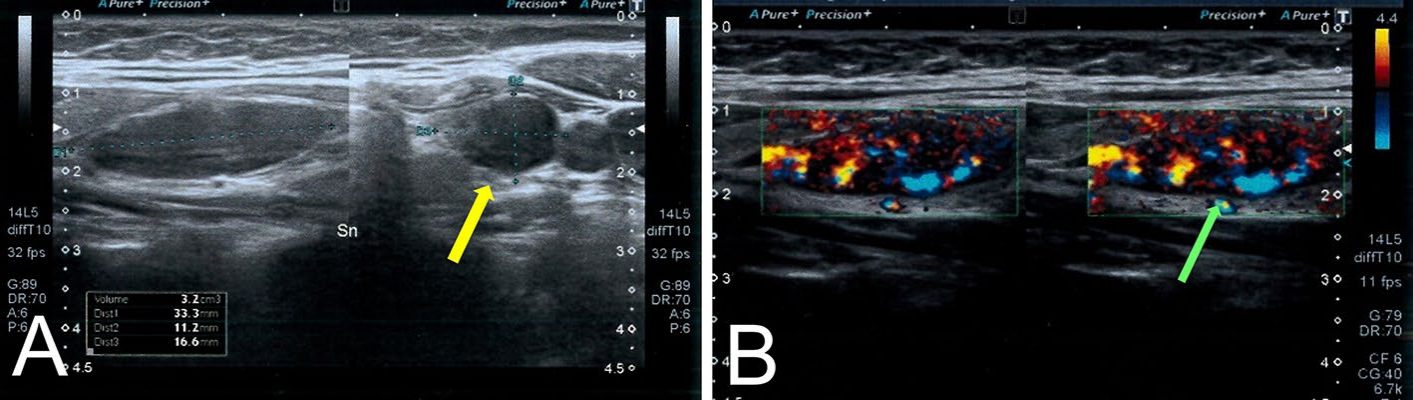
Ultrasound (A) showing hyperplastic parathyroid tissue in the lower left parathyroid lodge (yellow arrow) with contextual vascularization at color-power-Doppler (green arrow) (B)
